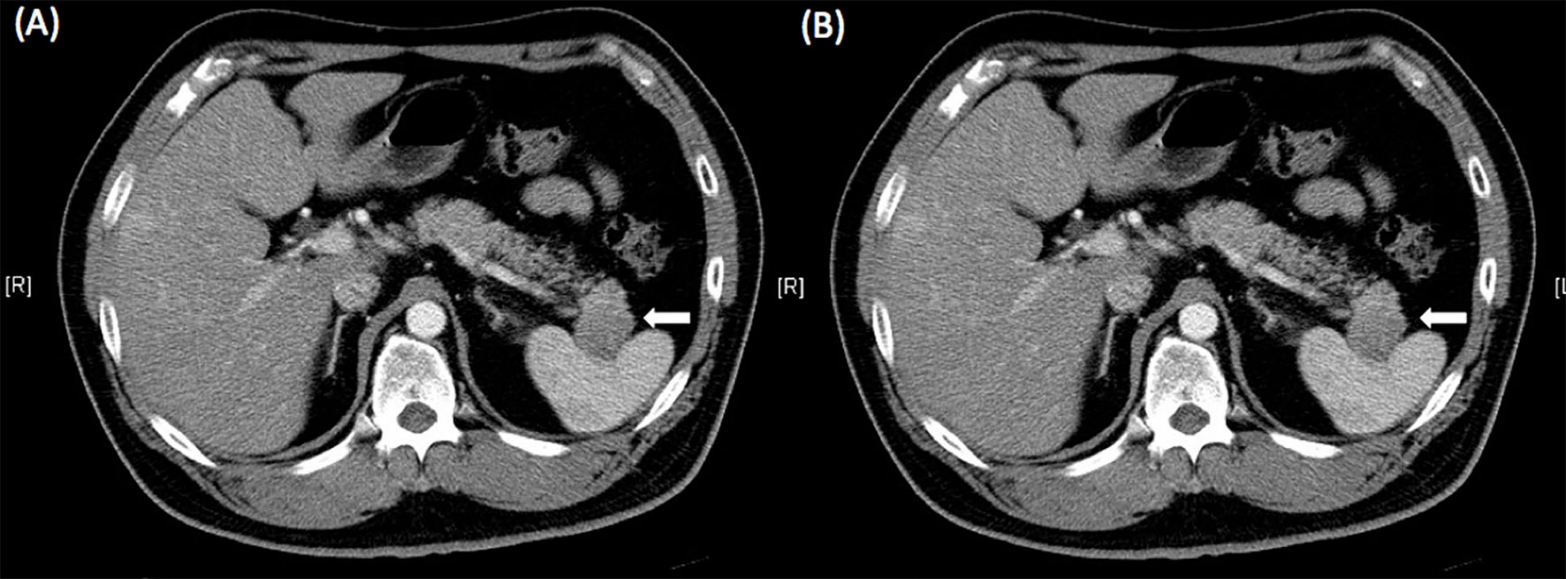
Abdominal computed tomography after 3 months of treatment with everolimus showed stationary pancreatic neuroendocrine tumors (A, white arrow) but decreasing liver metastasis nodule numbers (B), suggesting a partial response to everolimus.
